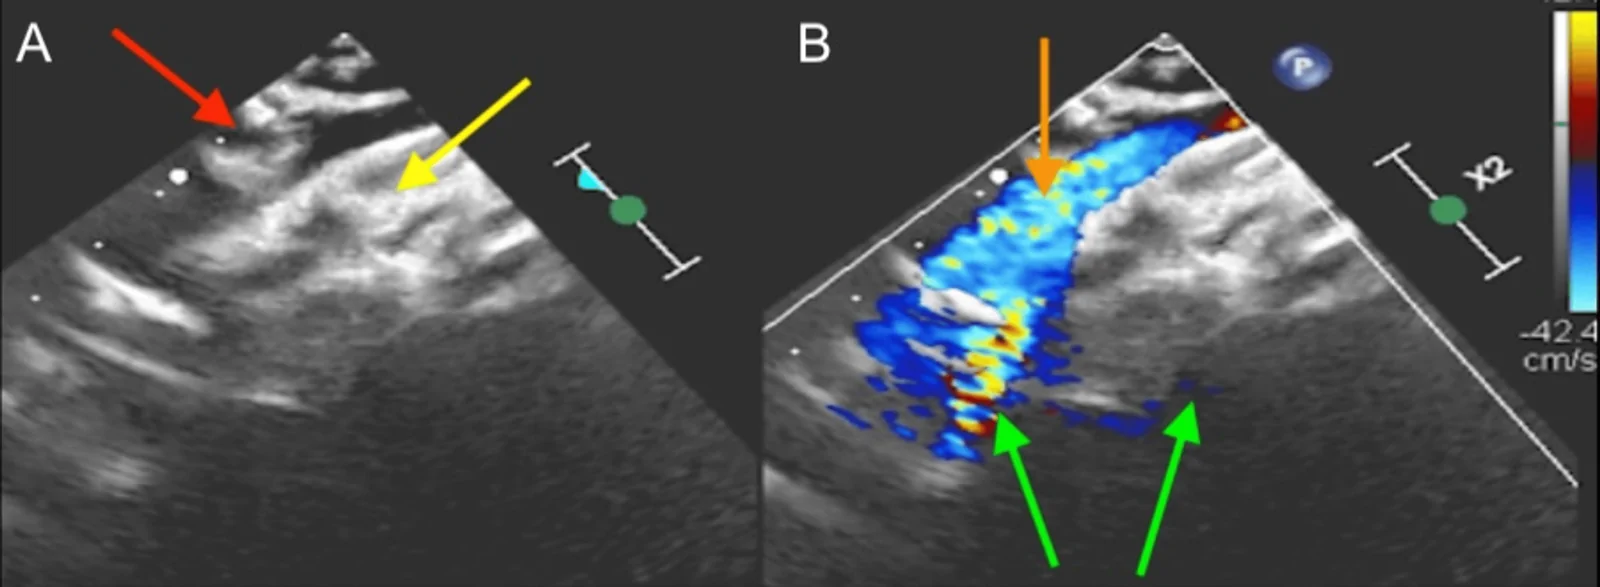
Transthoracic echocardiogram, suprasternal view following hemi-Fontan procedure. A) Grayscale imaging demonstrating the superior vena cava (SVC) (red arrow) and SVC-to-pulmonary artery anastomosis (yellow arrow). B) Color Doppler imaging demonstrating flow from the SVC (orange arrow) into the branch pulmonary arteries through the hemi-Fontan pathway (green arrows).
